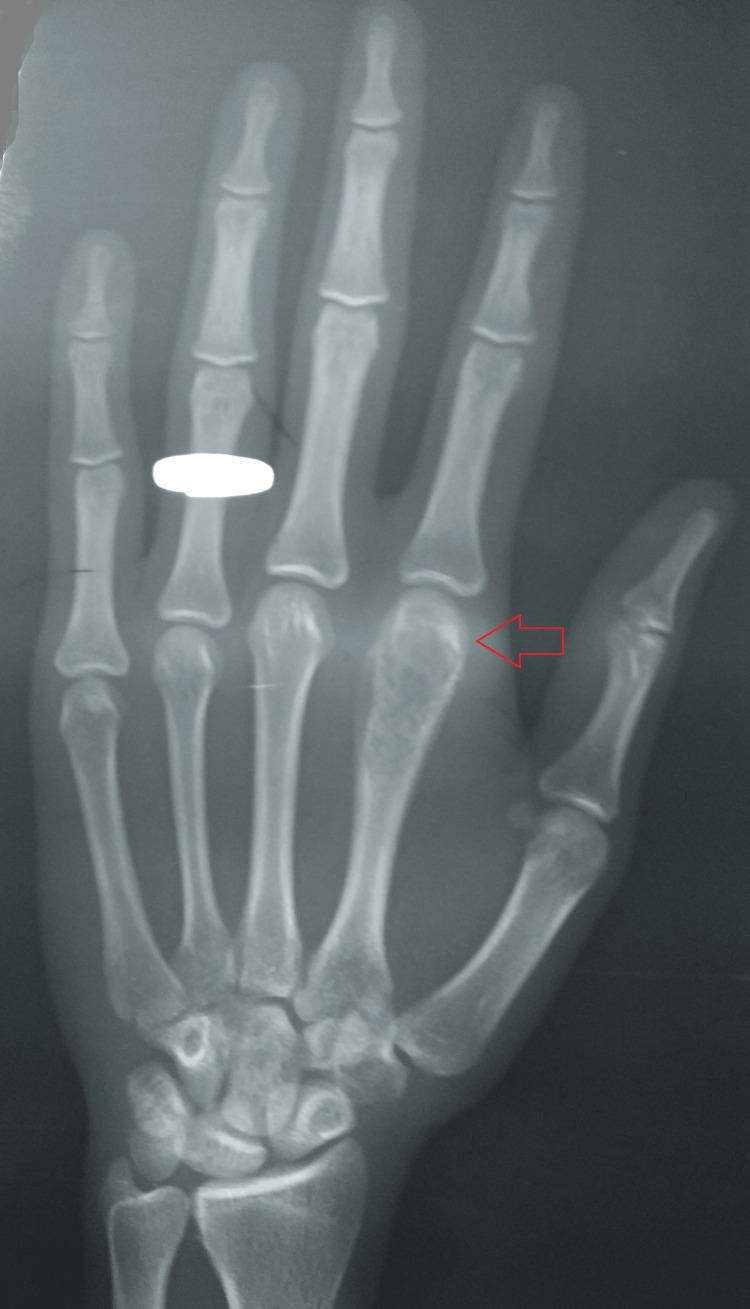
A plain radiograph-AP view of the hand showing a lytic lesion with slight cortical erosion and minimal periosteal reaction over the head of the second metacarpal of the left index finger AP - anteroposterior
